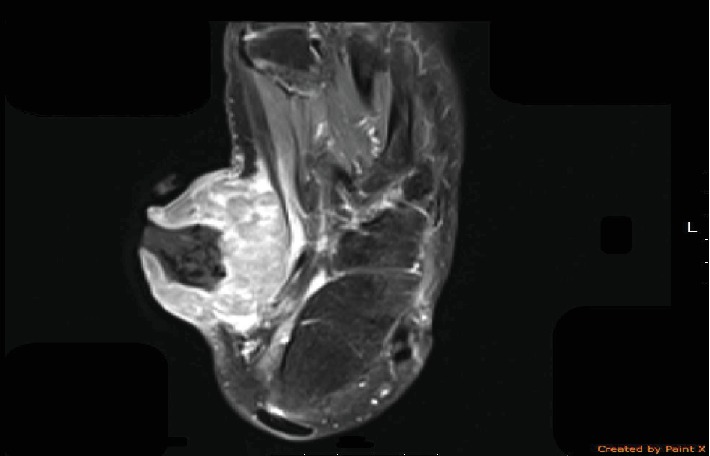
MRI of the left foot showing a large exophytic soft tissue mass in the medial side with necrotic center. The lesion involves the flexor hallucis longus and the abductor hallucis muscles.
